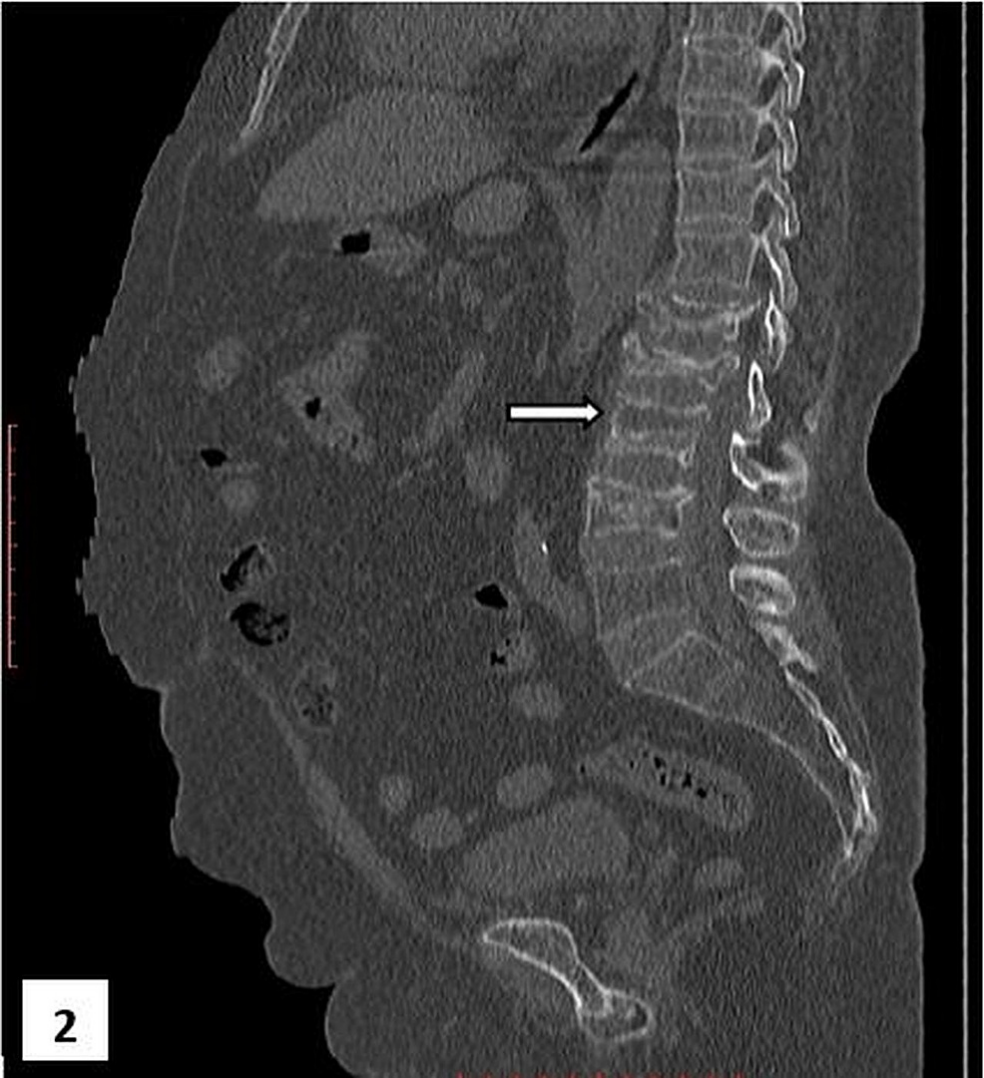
Computed tomography (saggital view) of the lumbosacral spine in the bone window The collapse of multiple vertebrae, a marked reduction in the vertebral body height, and a linear intraosseous cleft in L2 (arrow) are shown. L: lumbar.
